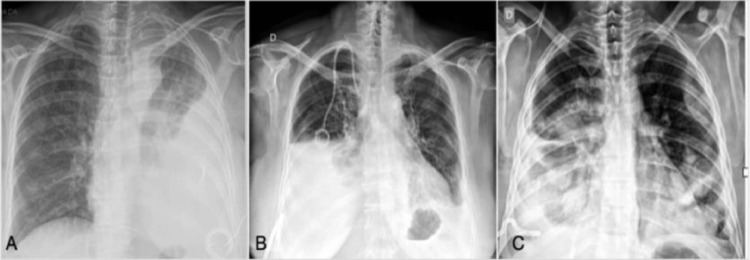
Classification of chest radiographs according to lung pleural expansion after drainage. Caption: (A) Lung pleural expansion less than 50% on the left side, (B) lung pleural expansion between 50‒70% on the right side, (C) Lung expansion more than 70% on the right side.
